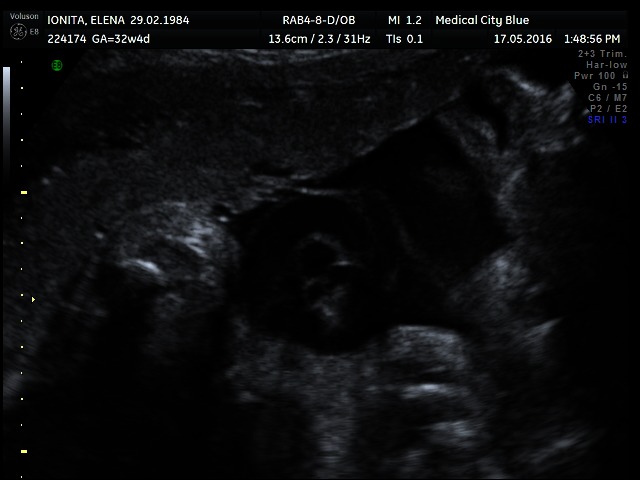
A hanging noose sign is seen on the 2-dimensional ultrasonography; a transverse section of the umbilical cord is surrounded by a loop of umbilical cord
